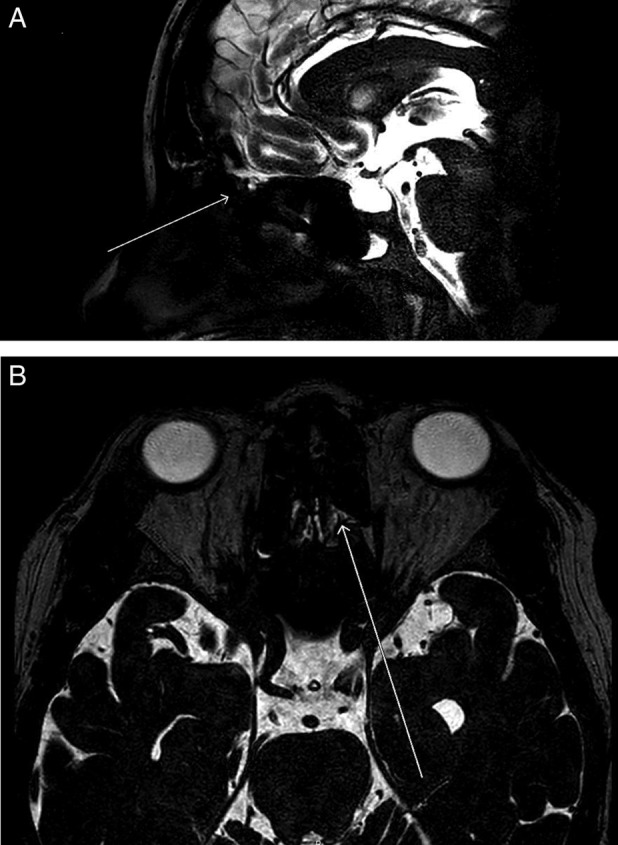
(A, B) Sagittal T2‐weighted magnetic resonance imaging scan demonstrating CSF leak from the anterior cranial fossa via the cribriform plate to the superior nasal cavity.
